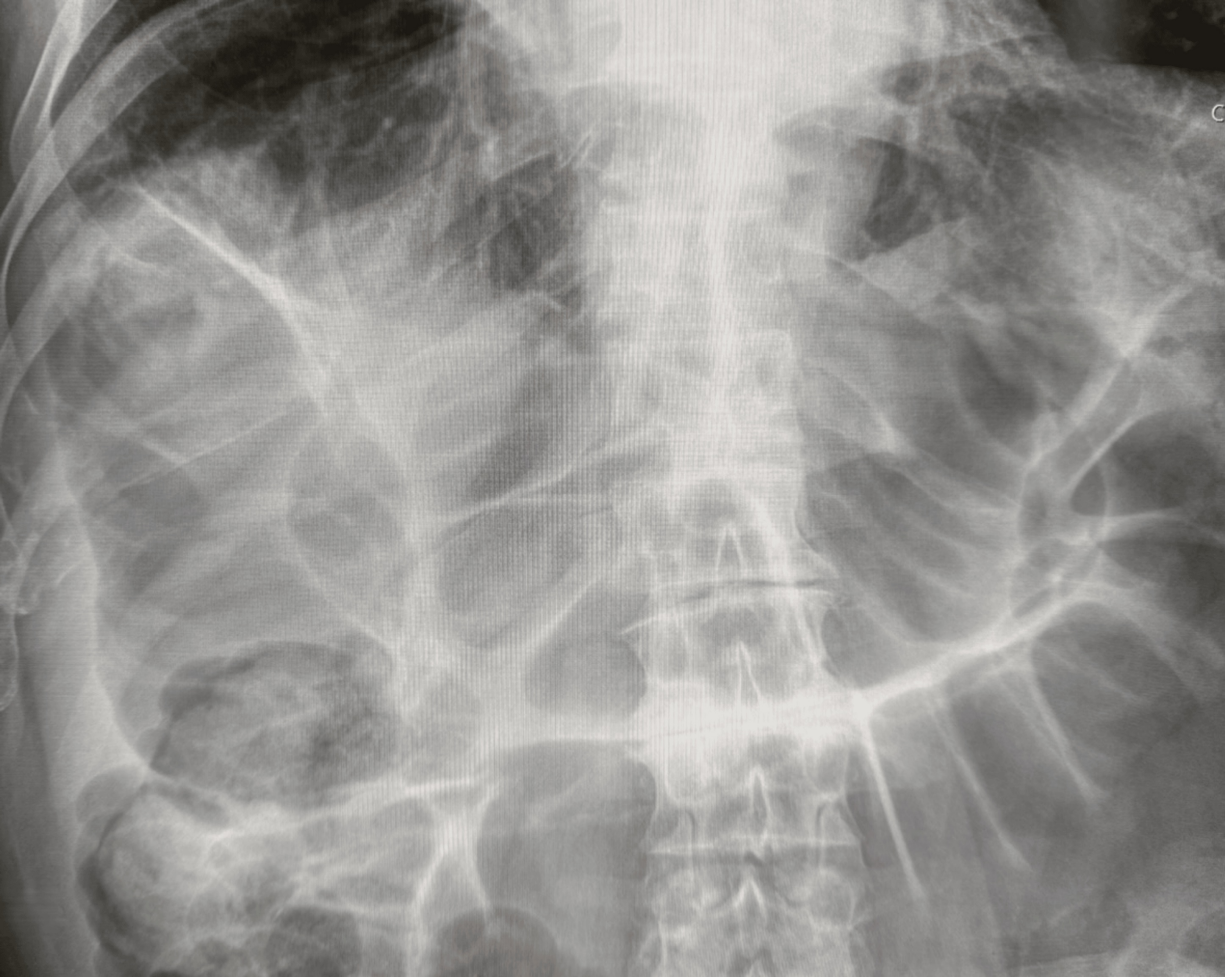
Kidney, ureter, bladder (KUB) radiograph at the rehabilitation hospital showed diffuse dilation of the large intestine without a clear transition point. The marked colonic distension is an important clue for Ogilvie's syndrome.
